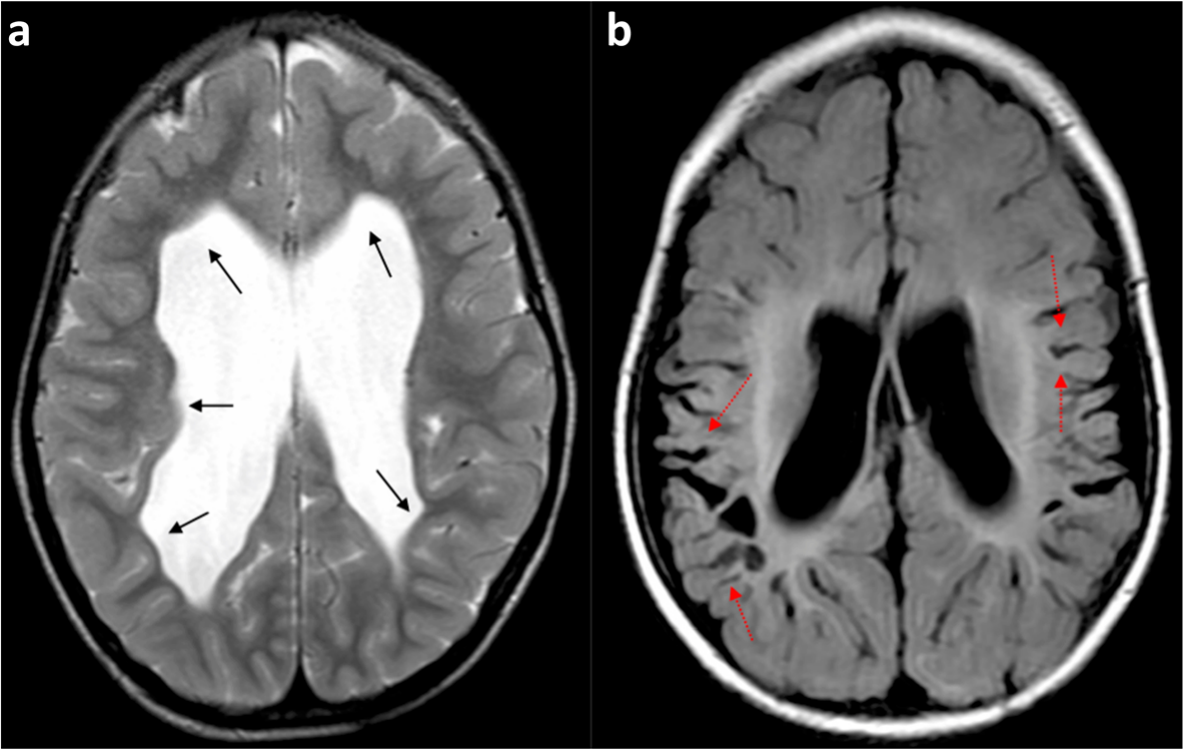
a, b Comparison of (a) Axial T2-weighted image showing periventricular leukomalacia (PVL) with predominantly periventricular white matter volume loss in a 6-year-old boy who sustained a global hypoxic-ischaemic injury as a premature neonate, compared with (b) axial FLAIR image showing predominantly subcortical white matter loss in a 1-year-old boy who sustained a severe partial-prolonged hypoxic-ischaemic injury at term gestation. In the patient born prematurely with PVL (a), there is such severe periventricular white matter volume loss that the cortical mantle at the sulcal depths abuts the ventricular ependymal edges, causing a ‘wavy’ ventricular margin (black arrows). In addition, the width of the sulci is of equal size at their depth as at their surface, with sulci and gyral surfaces remaining parallel. The patient born at term with partial-prolonged hypoxic-ischaemic injury (b) demonstrates preservation of some of the periventricular white matter substance (even though of abnormally high signal) and regular ventricular margins as the cortical mantle at the sulcal depth is separated from the ventricular ependymal edge. There is also ulegyria at the peri-Sylvian regions bilaterally (red dotted arrows)
